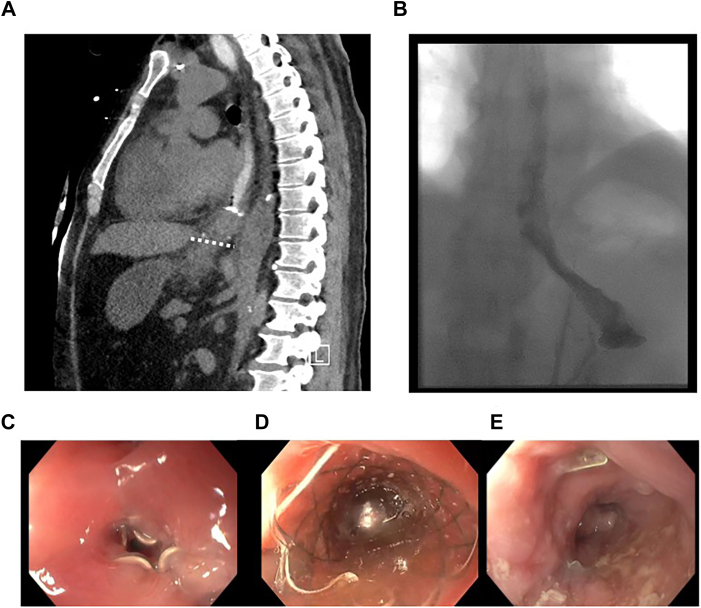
(A) Computed tomographic scan after emergency surgery with staples (dotted line demarcates placement of distal staples). (B) Esophagram showing restoration of flow after successful stenting. (C) Endoscopy with an exclusion staple line. (D) Endoscopy showing stent deployment through the staples. (E) Endoscopy after removal of the stent showing resolved and healed esophagus.
